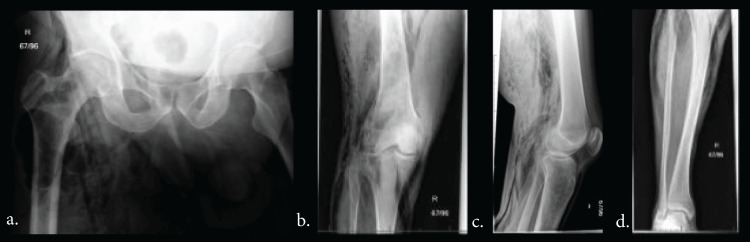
Plain film showing air in tissue planes a. Pelvic X-ray, b. right knee posteroanterior (PA) film, c. right knee lateral film, d. right lower limb PA film.
